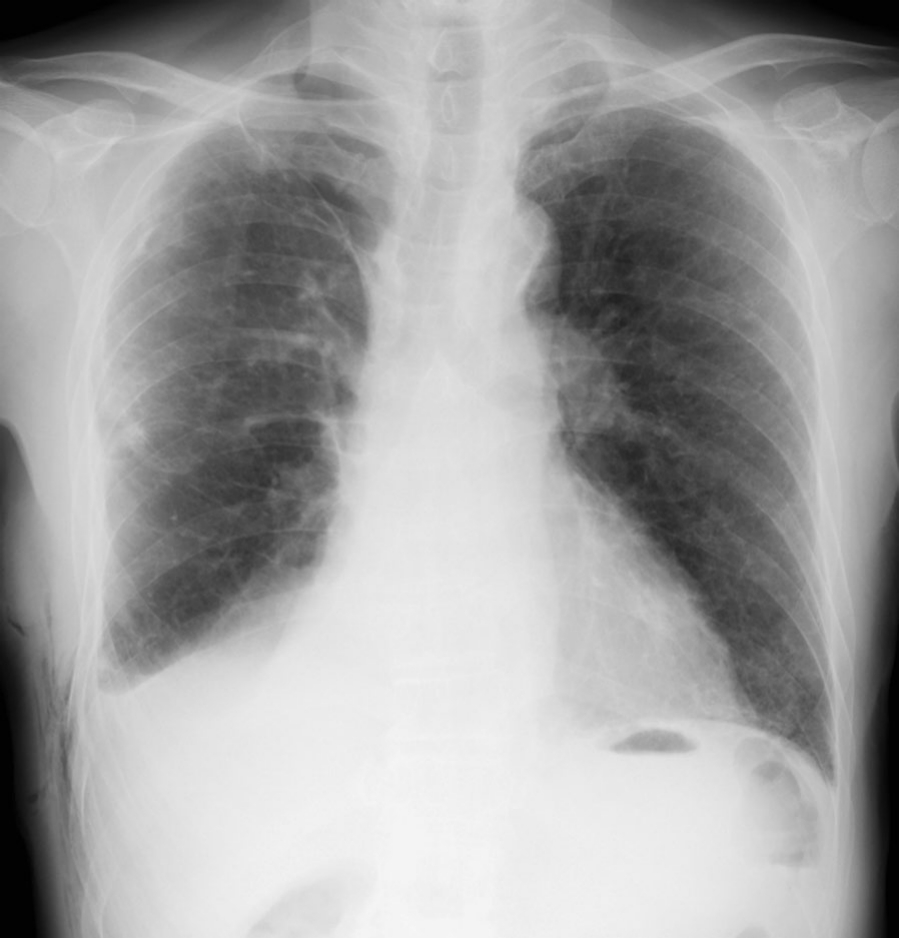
Chest X-ray of the patient before he was discharged from the initial hospitalization showing no problems after right lower lobectomy
